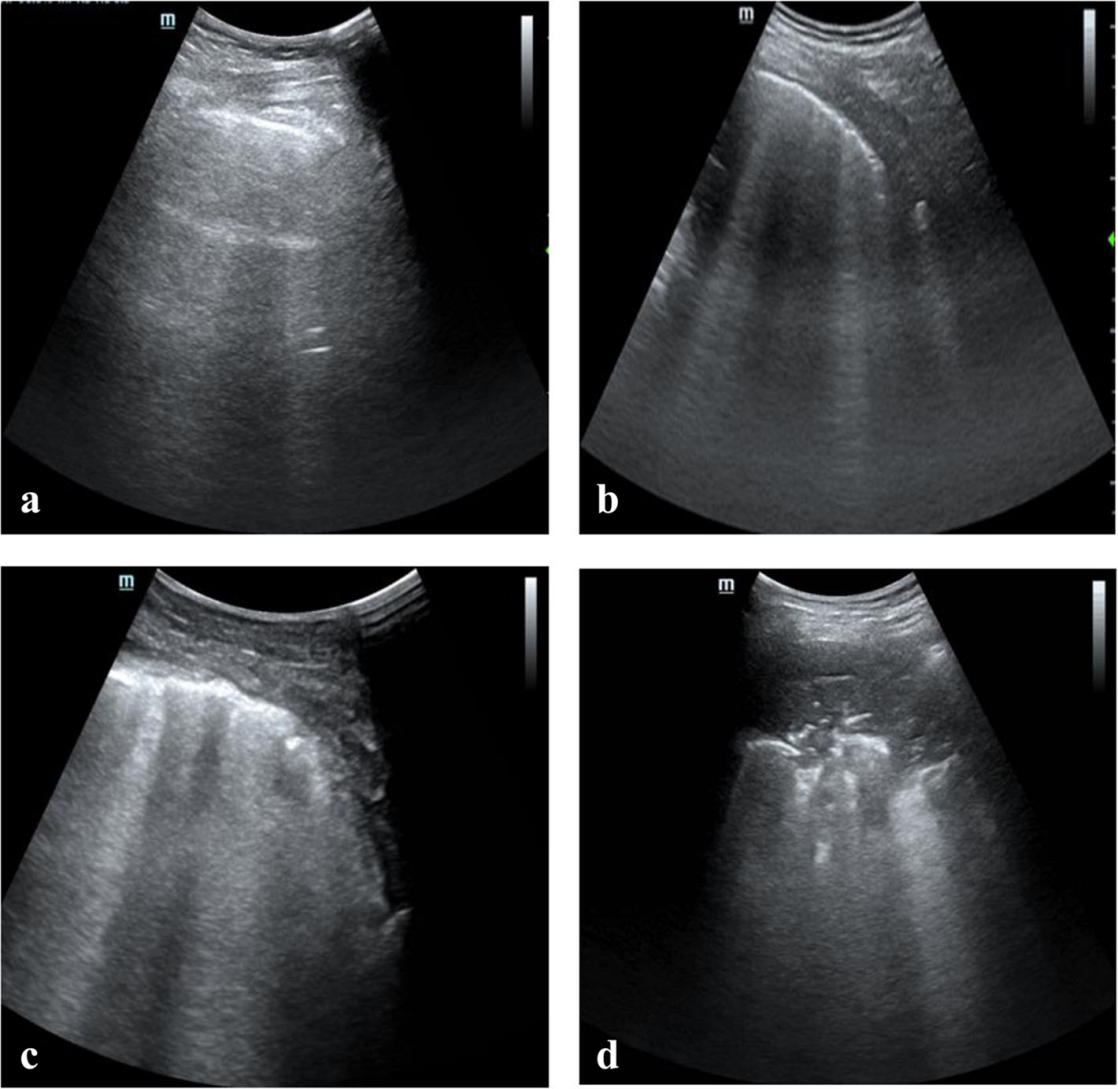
Lung ultrasound signs with different scores. a score 0, healthy lung, equidistant A-lines parallel to the sliding pleura; b score 1, moderate aeration loss, no fewer than 3 dispersive B lines originated from the pleura; c score 2, serious aeration loss, presence of coalescent B lines with irregular pleura; d, score 3, absolute aeration loss, subpleural consolidation
